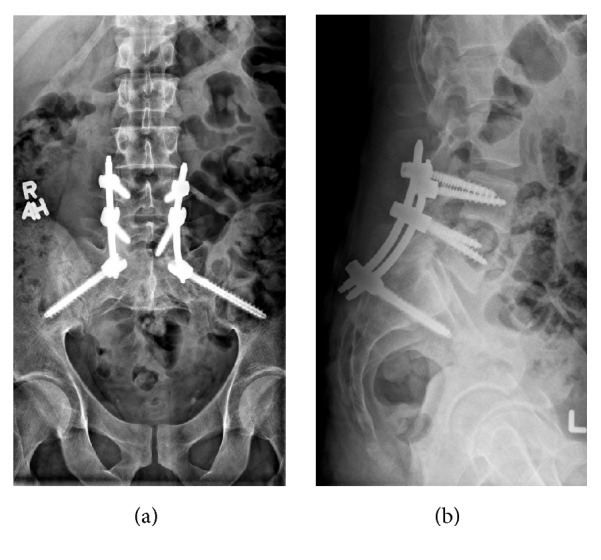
AP (a) and lateral (b) radiographs of patient seen in Figure 1 at 16 months postoperatively treated with percutaneous fixation. Note the resolution of kyphotic deformity with consolidation of fracture lines.
